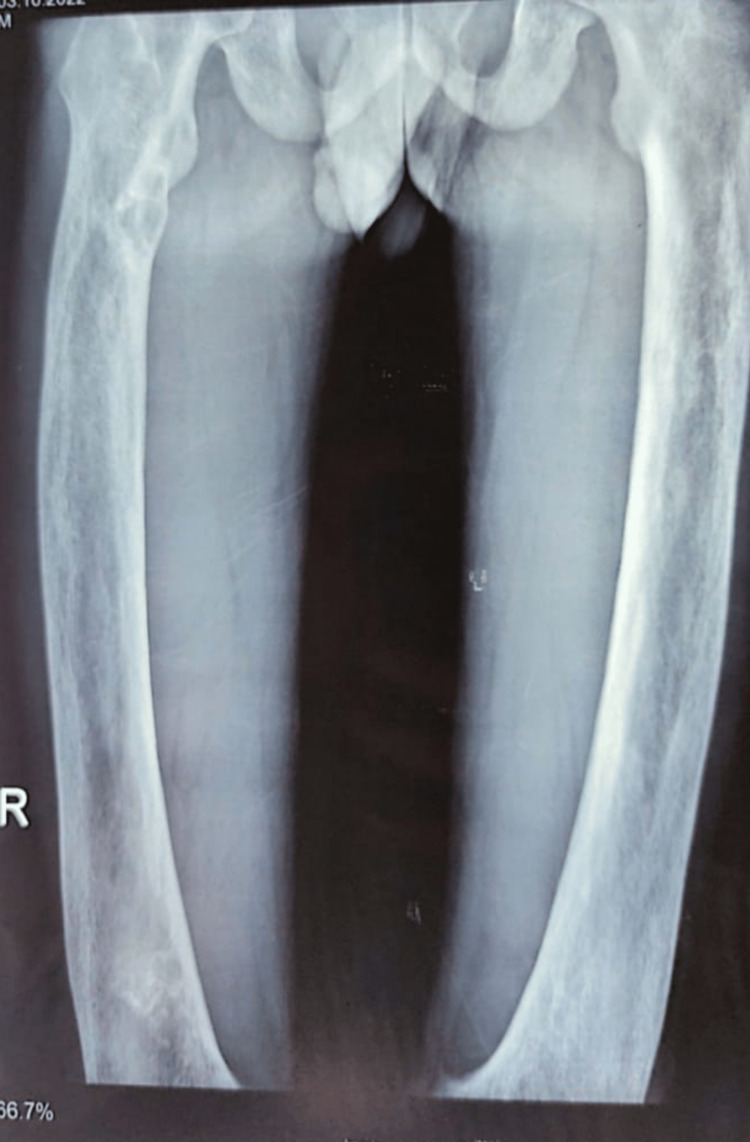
Radiograph of long bones showing cortical thickening and alternating osteolysis and osteosclerosis. There is lateral bowing of the femurs and a characteristic "blade of grass" sign present.
